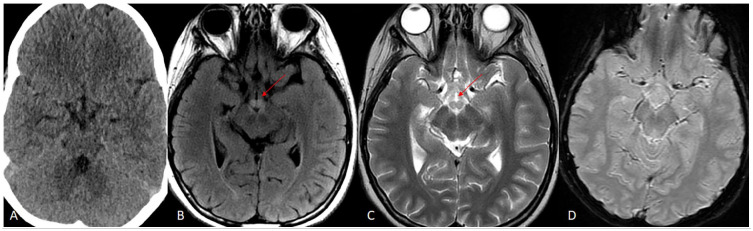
Initial CT scan without contrast is negative. Subsequently performed MRI brain on the same day demonstrates T2/FLAIR hyperintense signal in bilateral mammillary bodies (images A and B; red arrows). No associated gradient blooming to represent hemorrhage.
